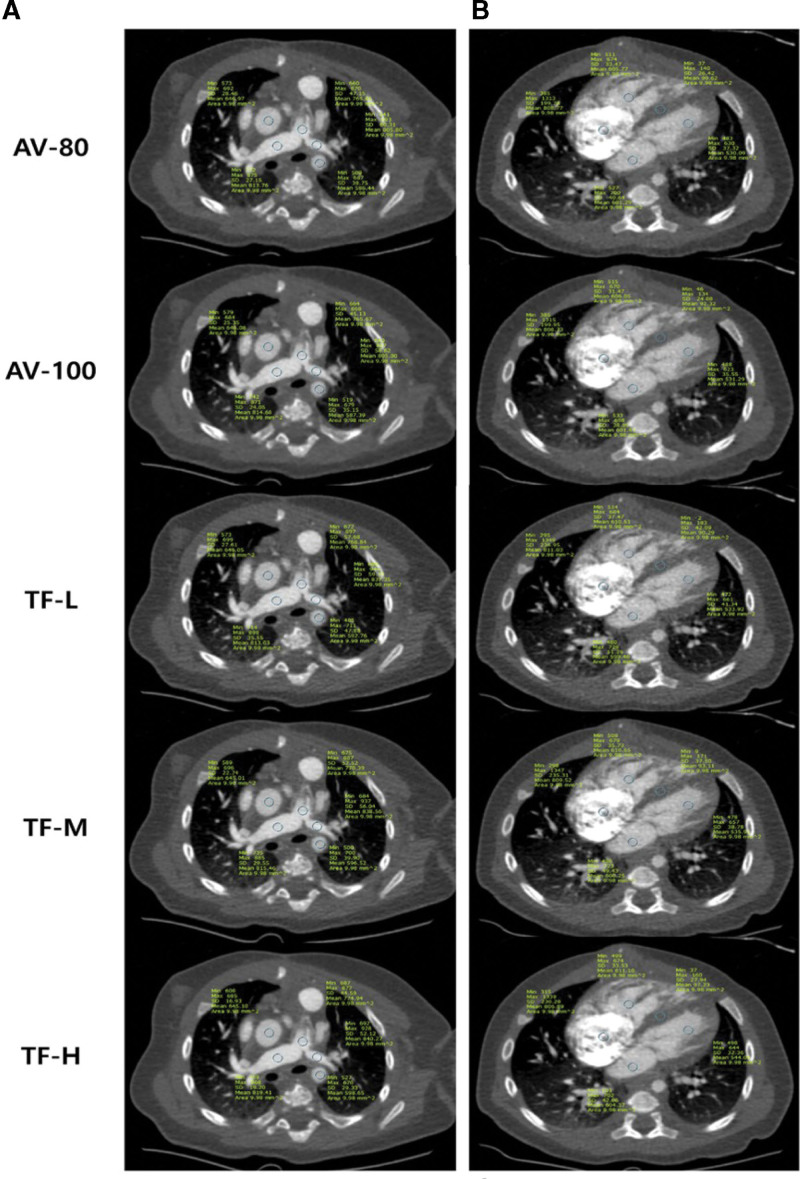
Axial contrast-enhanced CT images from a 1-month-old female patient with atrial and ventricular septal defect, at the visible level of the pulmonary trunk, right pulmonary artery, left pulmonary artery and 4-chamber view. ROI was measured for each reconstruction image of (a) AV-80, (b) AV-100, (c) TF-L, (d) TF-M, and (e) TF-H, then a total of 10 structures, namely, the left atrium, left ventricle, right atrium, right ventricle, pulmonary trunk, left pulmonary artery, right pulmonary artery, ascending aorta, descending aorta, and interventricular septum, were obtained. AV-80 and AV-100 = ASIR-V with blending factors of 80% and 100%, respectively; TF-L, TF-M, and TF-H = TrueFidelity with low, medium, and high strength levels, respectively. CT = computed tomography, ROI = region of interest.
